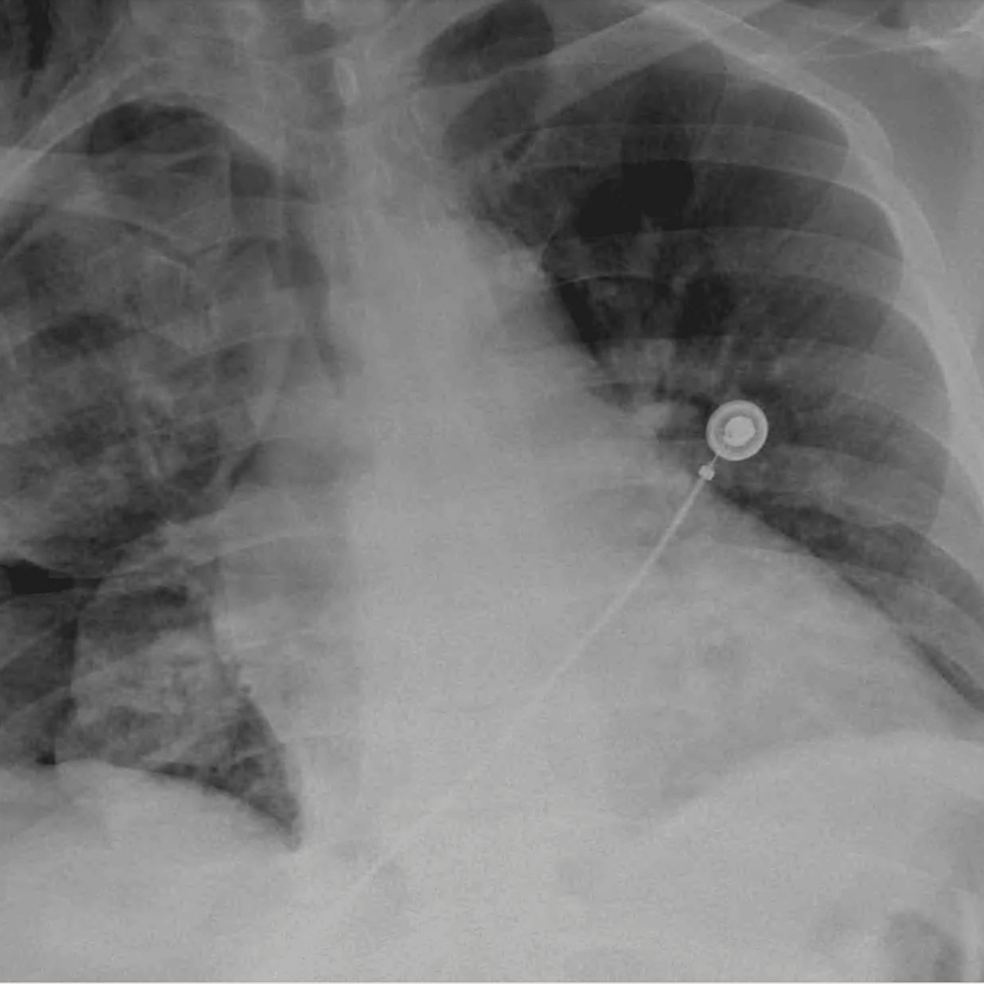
Chest x-ray post thoracocentesis.
